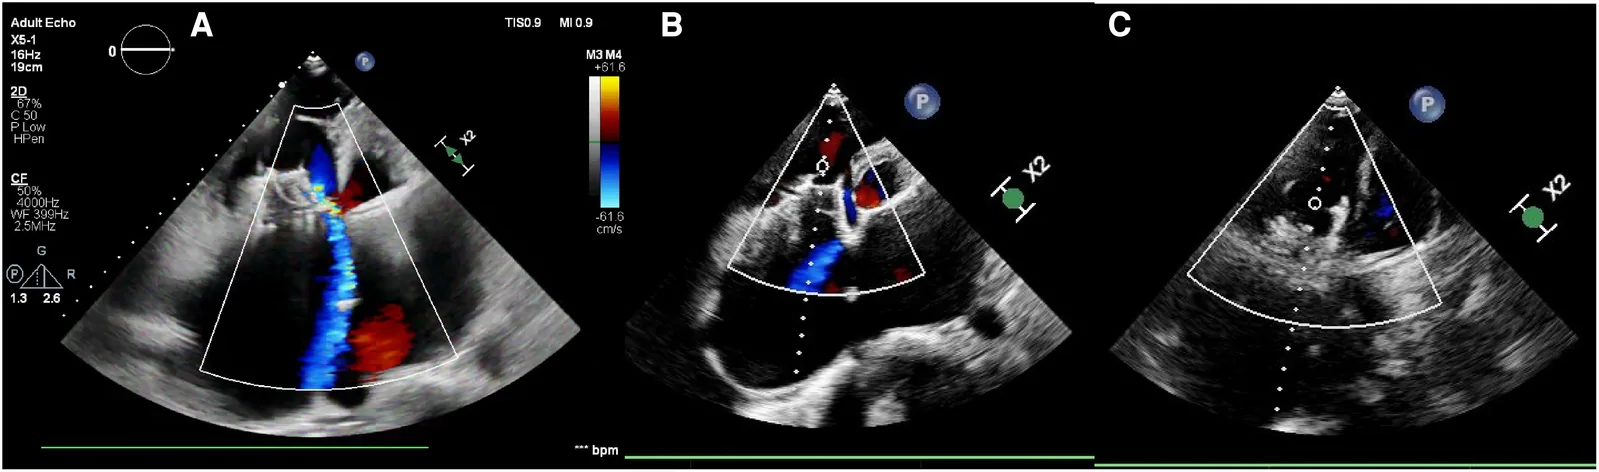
Echocardiographic follow-up after LuX-Valve Plus implantation. (*A*) Early postprocedural colour Doppler echocardiography showing moderate residual tricuspid regurgitation (2+). (*B*) At 1 month, the prosthetic position and haemodynamics were stable, with no obvious transvalvular regurgitation and only trivial paravalvular leak. (*C*) At 6–7 weeks, the prosthetic position and haemodynamics remained stable, with no obvious transvalvular regurgitation or paravalvular leak.
